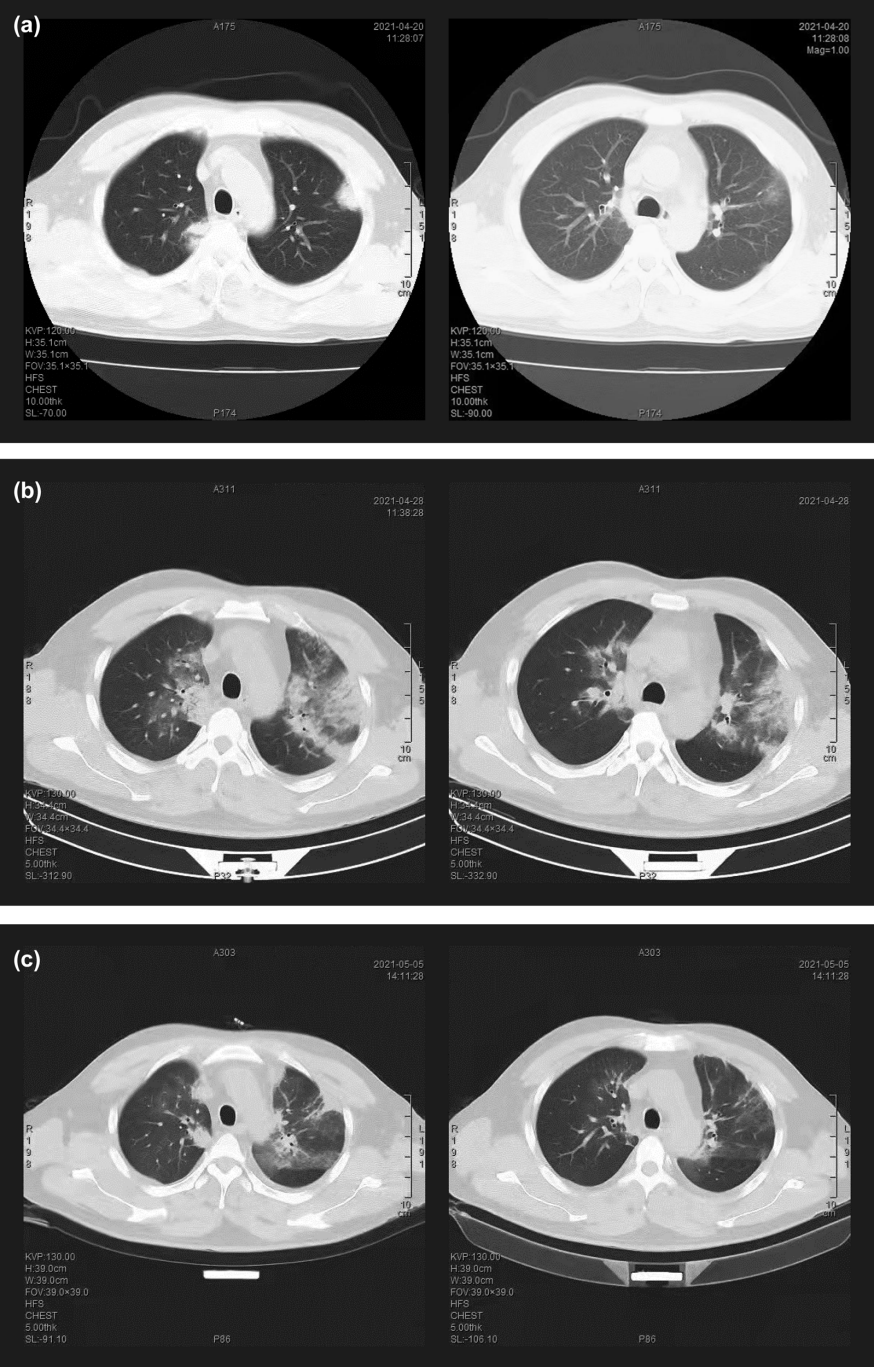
Chest CT scan during admission. (a) The chest CT images on April 20, 2021 showed nodules in the upper lobes of the lungs and a little halo sign. (b) The chest CT images on April 28, 2021 showed a range of flocculent high-density shadow increased in the lobes of bilateral pulmonary, peripheral halo sign diffused, and the lesions progressed in contrast to the images on April 20, 2021, especially the whole upper lobe of the left lung showed ground-glass opacity. (c) The chest CT images on May 5, 2021 showed that lesions are mostly absorbed and pneumonia was significantly improved.
